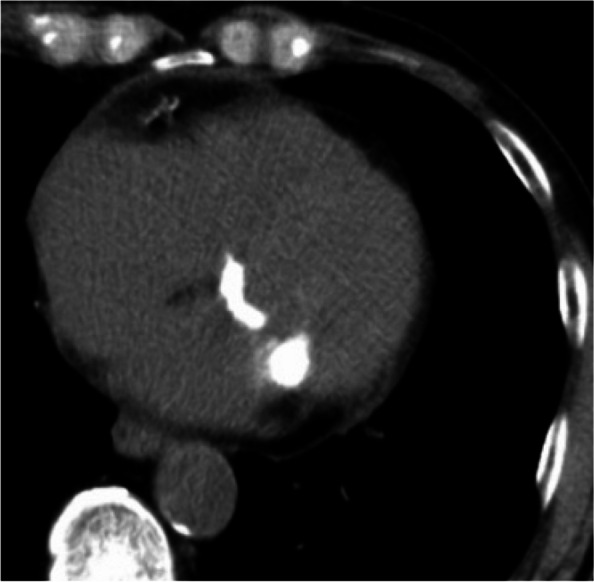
Computed tomography revealing mitral annular calcification
